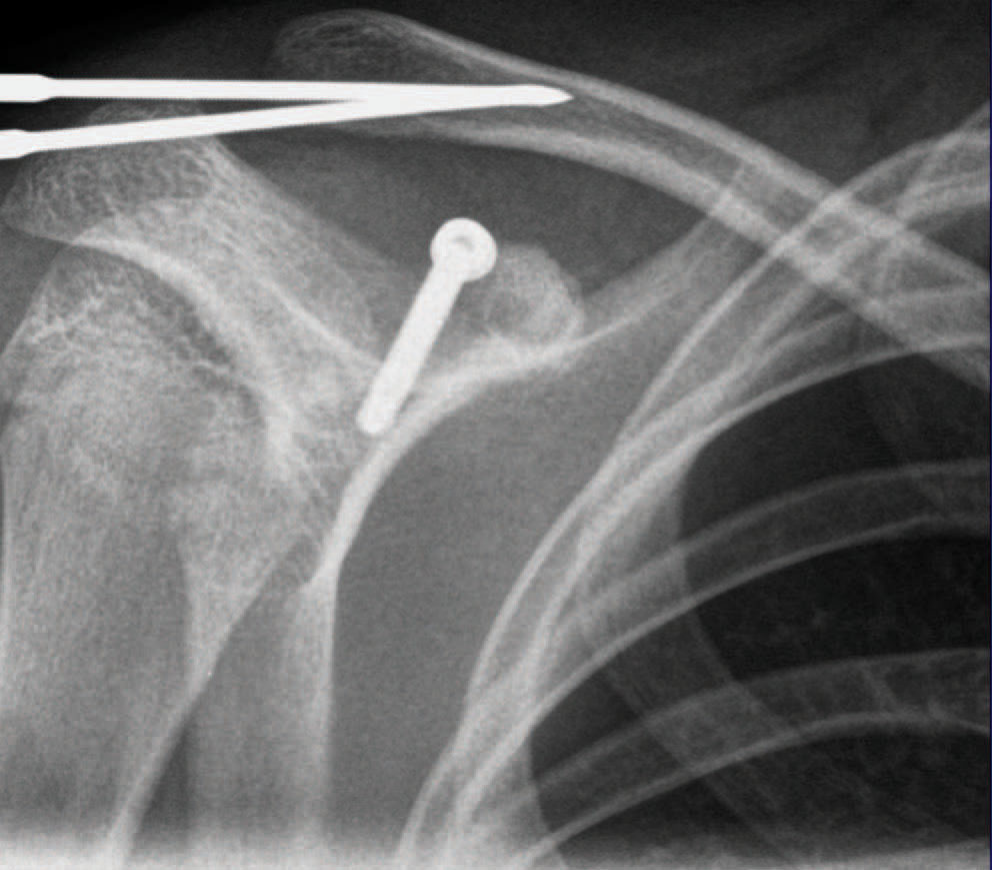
Post-operative radiograph anteroposterior of the right shoulder.
